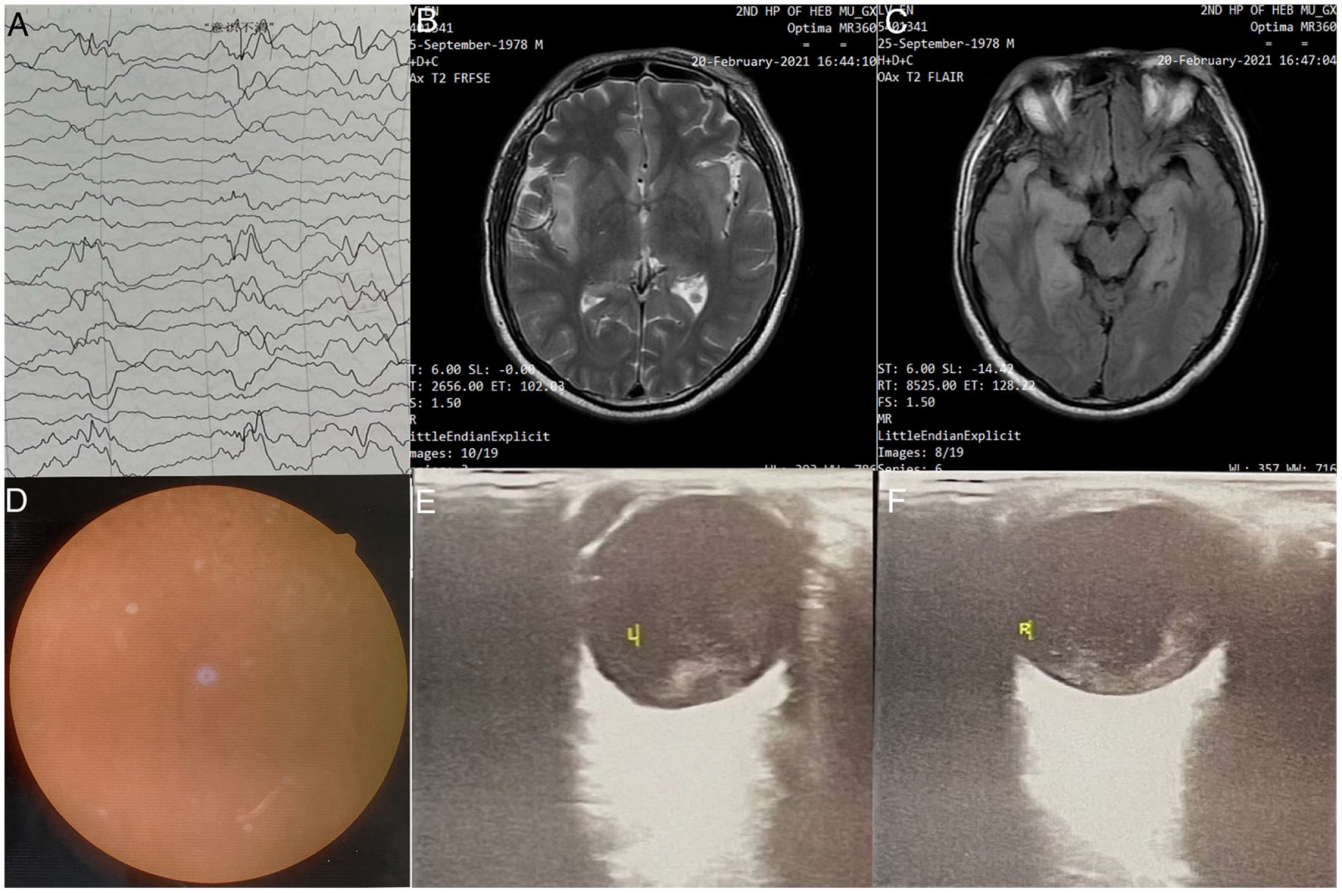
(A) Electroencephalography findings: Severe abnormal diffuse mixed slow waves with a lot of fast waves, intermittent low-voltage waves, lasting about 1s, diffuse 1-2Hz irregular s activity, left sharp waves and sharp slow waves are emitted from the front side of the head; (B) Head MRI - T2 FRFSE: Bilaterally symmetrical abnormal signals are observed in the anterior cingulate gyrus; (C) Head MRI - T2 FLAIR sequence: Symmetrical abnormal signals of insula and fronto temporal lobes; (D) Fundus examination: Both eyes have clear cornea with no hyperemia, anterior chambers, pupil diameter of ~3mm, slow reflection of light; (E) Ophthalmic ultrasound-L: (1) Severe vitreous opacity; (2) Thickening of the posterior wall of eyeball; (3) Thickening of the four rectus muscles of the eyes; (F) Ophthalmic ultrasound-R: (1) Severe vitreous opacity; (2) Thickening of the posterior wall of eyeball; (3) Thickening of the four rectus muscles of the eyes. The ring of the right eye is intact, the lens wave is visible, and the dark area can be seen in the vitreous body. There is medium to high amount of diffuse flocculent weak echo and cluster echo. The posterior wall of the ball is thickened and slightly rough. The thickness of the superior rectus, external rectus, inferior rectus, and medial rectus is ~5.9, 5.6, 5.6, and 6.4mm, respectively.
